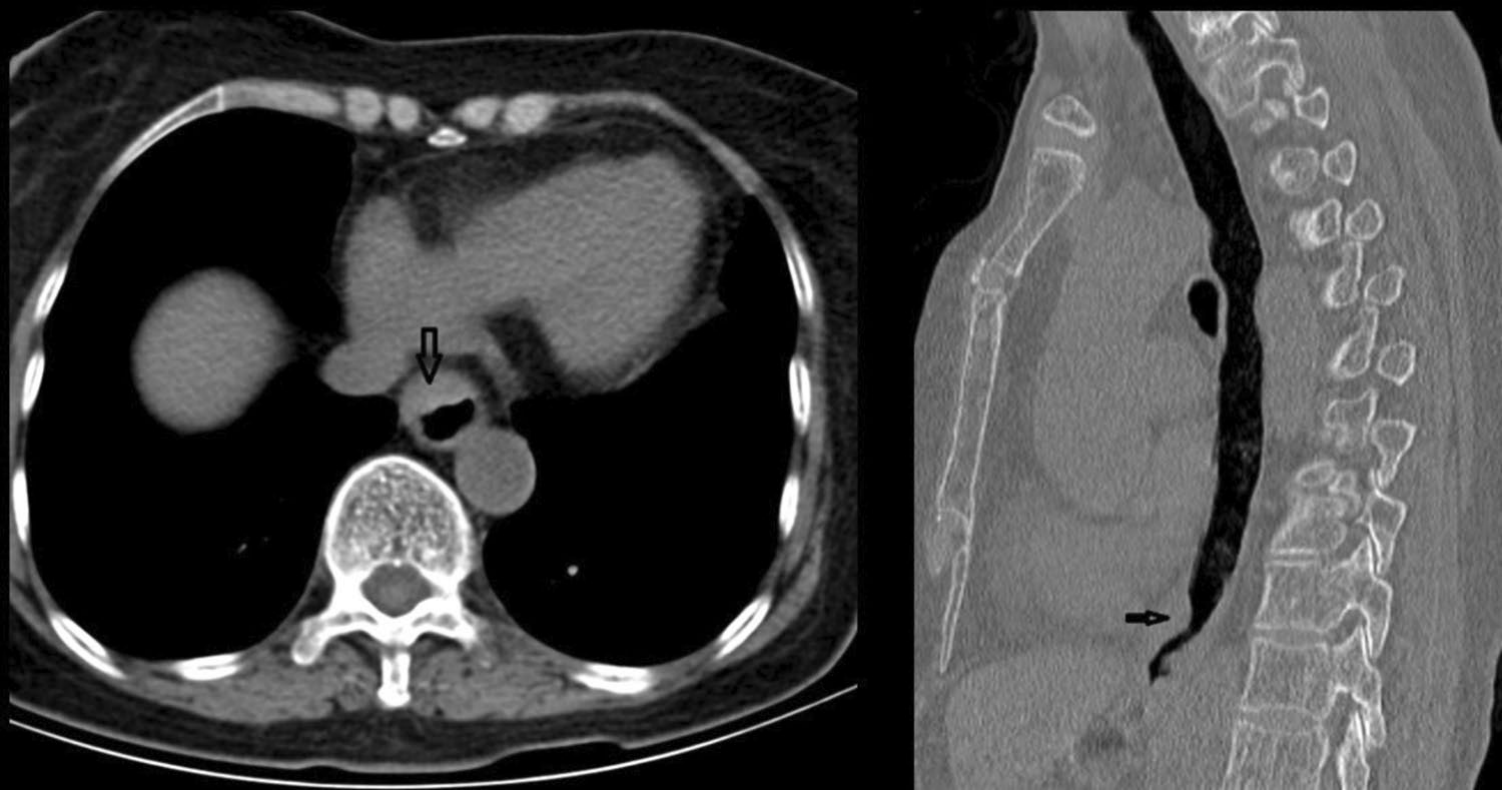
Computed tomography (CT) image of a filling esophagus with gas-producing powder. A CT axial image of esophageal filling with gas powder, showing a thickening of the esophageal wall. B Sagittal view of the filling gas powder of the esophagus, showing thickening of the esophageal wall and narrowing of the lumen
